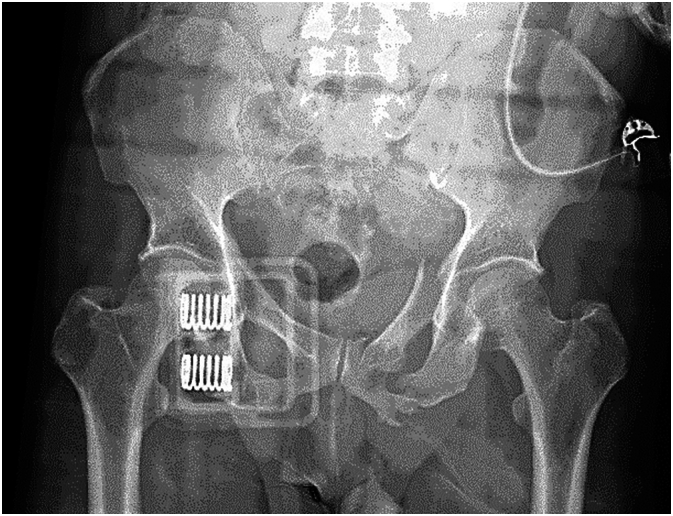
Pelvis X-ray during resuscitation, demonstrating left rami fractures and bladder displacement to the right. A pelvic binder is in situ.
